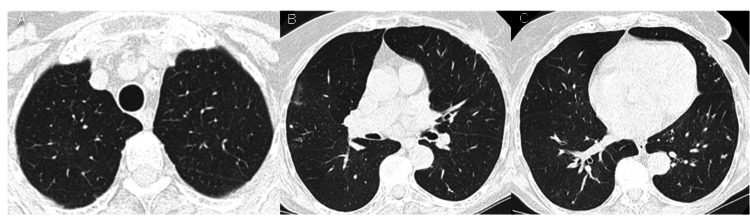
Chest CT 11 months after hospital discharge. Regressing pulmonary consolidations with corticotherapy.
